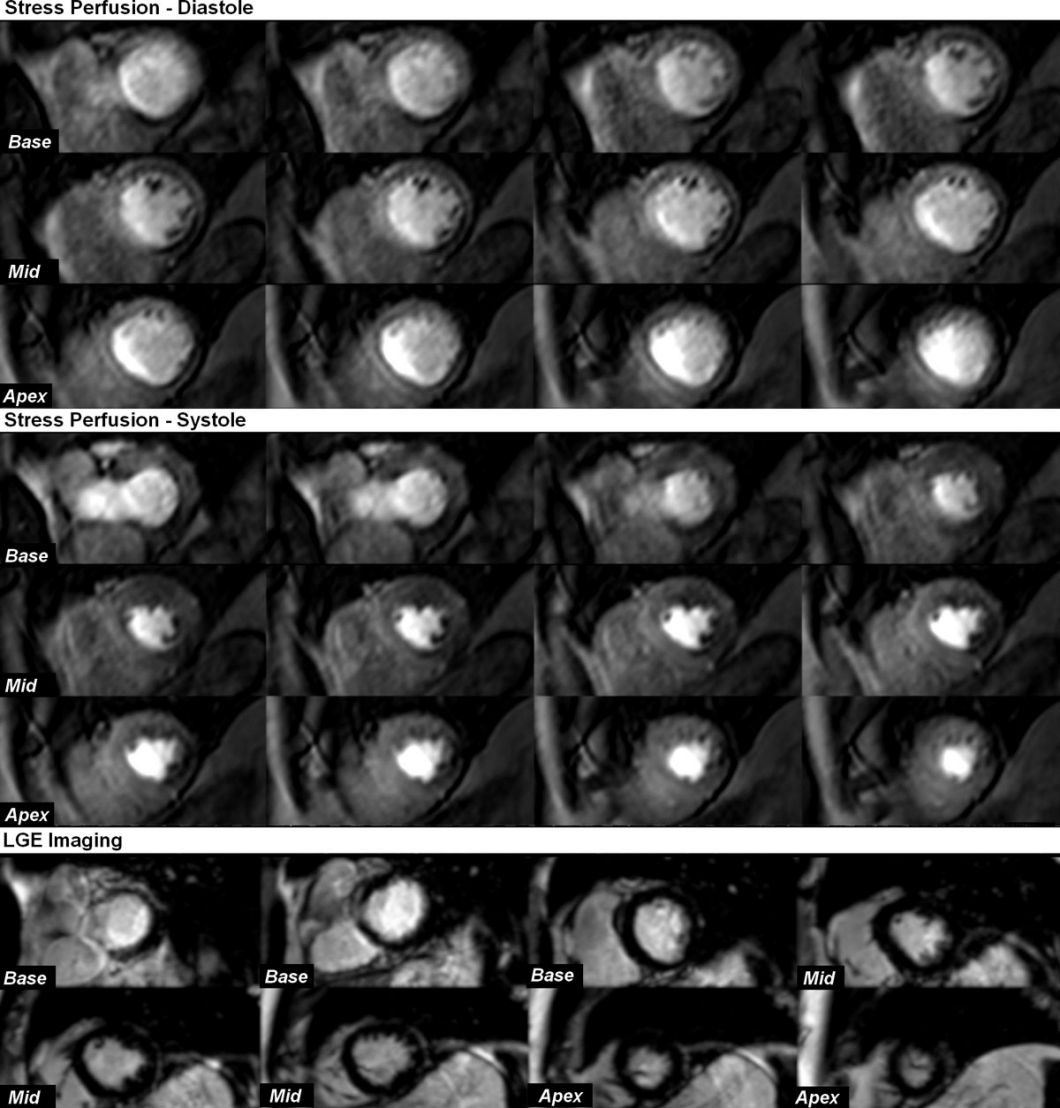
This example shows 3D-perfusion-CMR in a 75-year-old man presenting with angina. Stress-induced perfusion defects are seen infero-laterally from base to apex and antero-laterally from mid-ventricle to apex in both diastole and systole. However, perfusion defects are difficult to discern from dark-rim artifact in diastole and are more clearly delineated with systolic acquisition. Late-gadolinium enhancement imaging did not reveal any previous myocardial infarction. X-ray coronary angiography revealed 80% stenosis of a large diagonal branch and significant proximal disease in a large dominant left circumflex artery.
